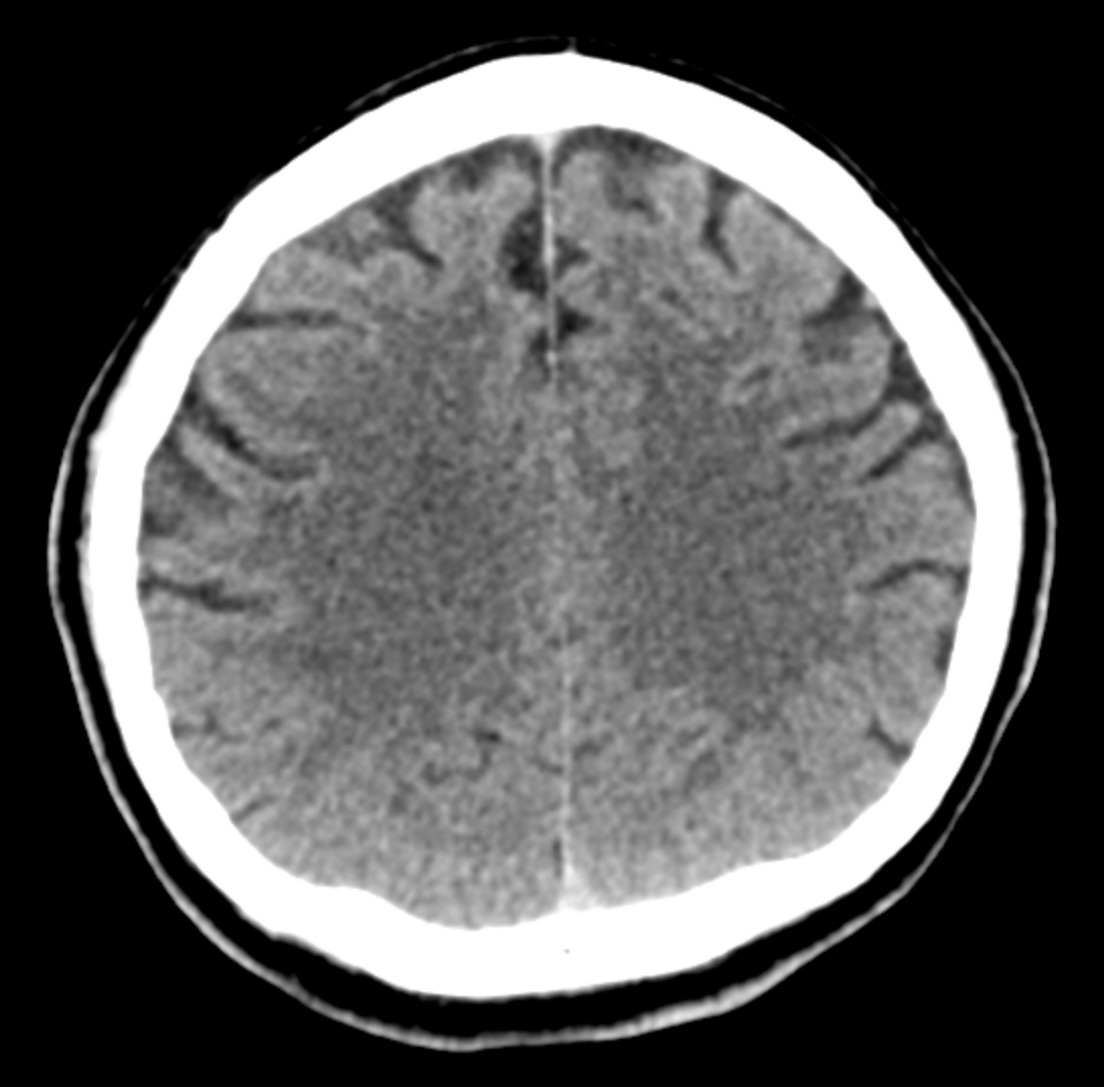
A repeat non-contrasted computed tomography scan of the brain 15 days after the index event revealed complete resolution of contrast material from the occipital lobes.
